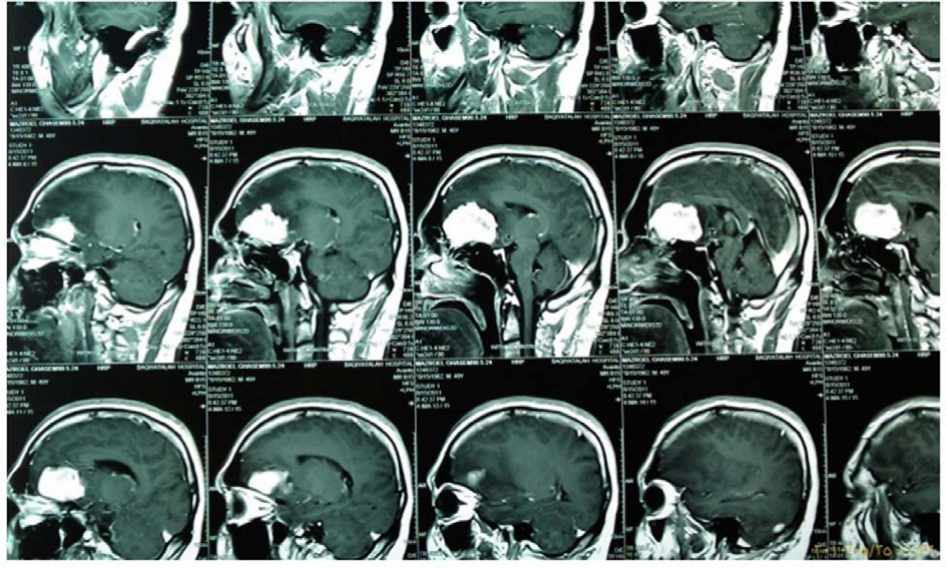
Brain magnetic resonance imaging shows frontal lobe meningioma
